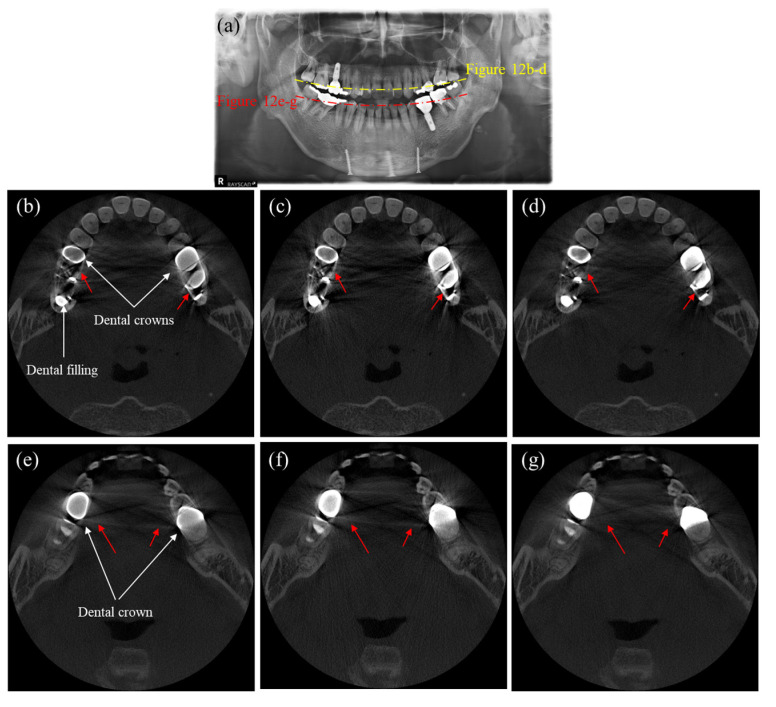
Images of a patient having multiple dental fillings and dental implants. (a) The panoramic X-ray image, (b,e) the uncorrected CT images, (c,f) the CT images corrected by the DSC method, and (d,g) the CT images corrected by the proposed method. (Contrast window is [−1117~8564] in Hounsfield Unit).
